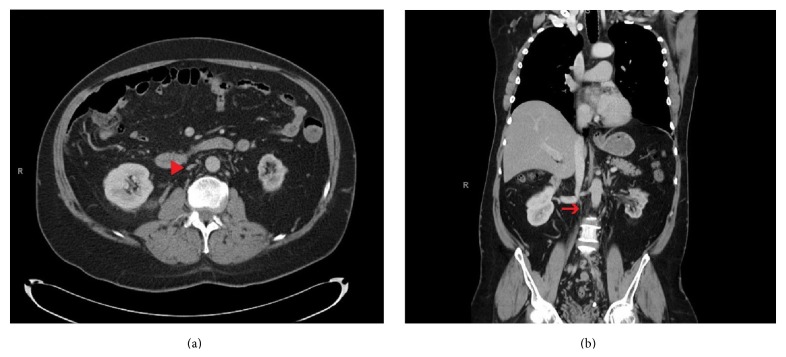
(a) Axial CT of the abdomen shows fibrosed infrarenal IVC (arrowhead). (b) Coronal CT of the abdomen shows thread-like fibrosis of the infrarenal IVC (arrow); note the presence of the IVC proximal to the renal veins.
